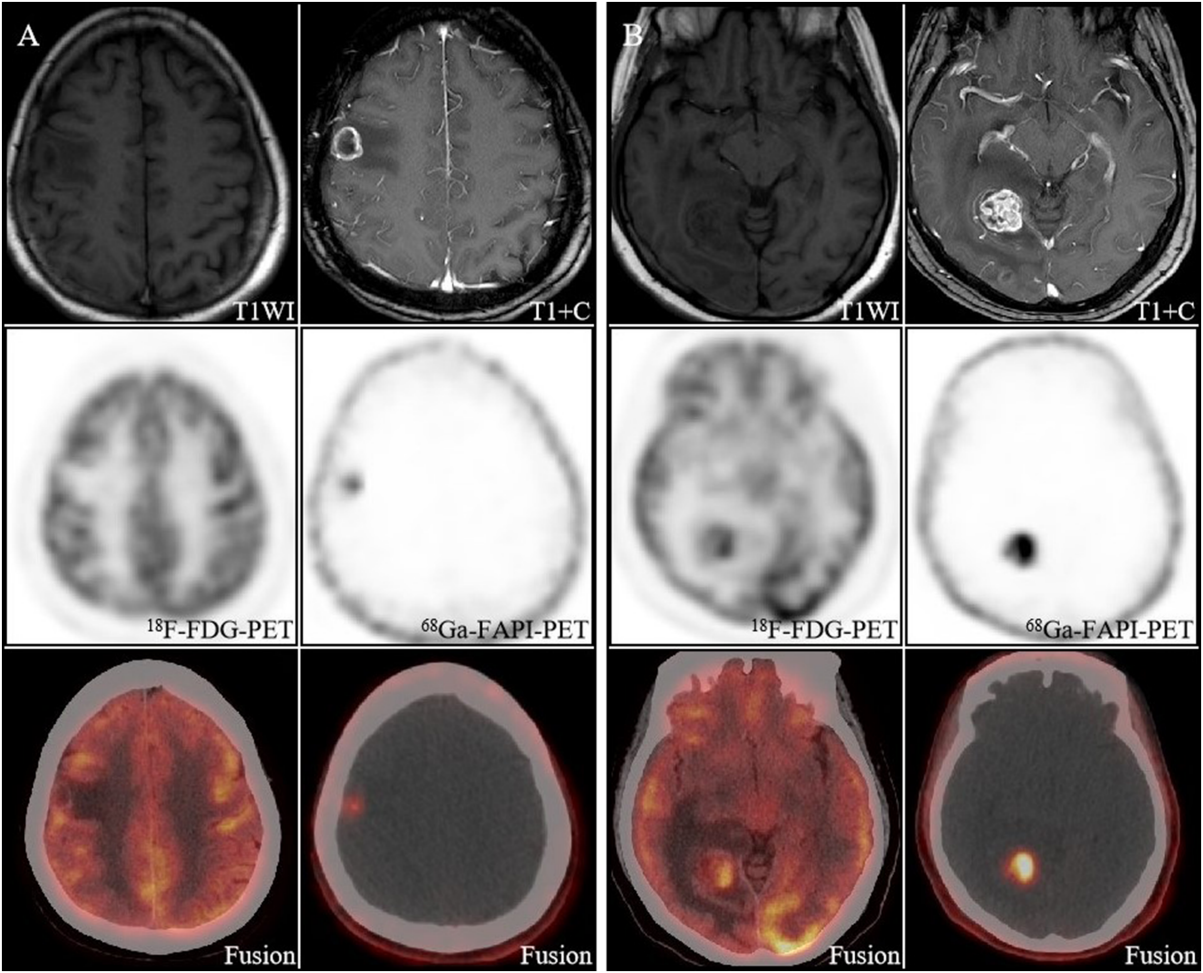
A 59-year-old woman with newly diagnosed brain metastases from lung cancer. Both the right parietal lobe lesion (A) and the right occipital lobe lesion (B) had morphological changes, with increased uptake of [^68^Ga]FAPI and a high TBR (38.3, 115), whereas on [^18^F]FDG PET/CT the lesions did not show as well as [^68^Ga]FAPI PET/CT.
